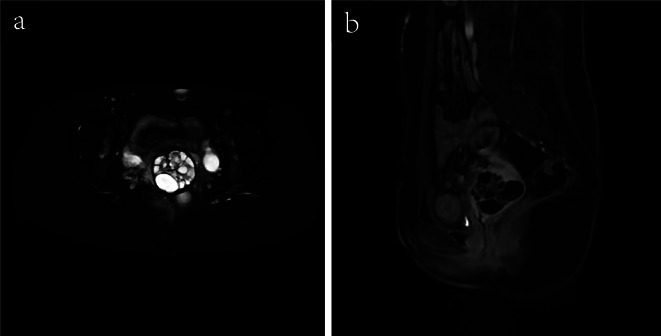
MRI examination of cervical lesions. (a) Cross-sectional plane on the T2WI fat suppression sequence exhibits an enlarged cervix with multilocular space-occupying lesions of various sizes abutted through irregular septae. (b) The sagittal plane of contrast-enhanced MRI reveals the noticeably enhanced cyst walls and septae. *Abbreviation* MRI, magnetic resonance imaging
